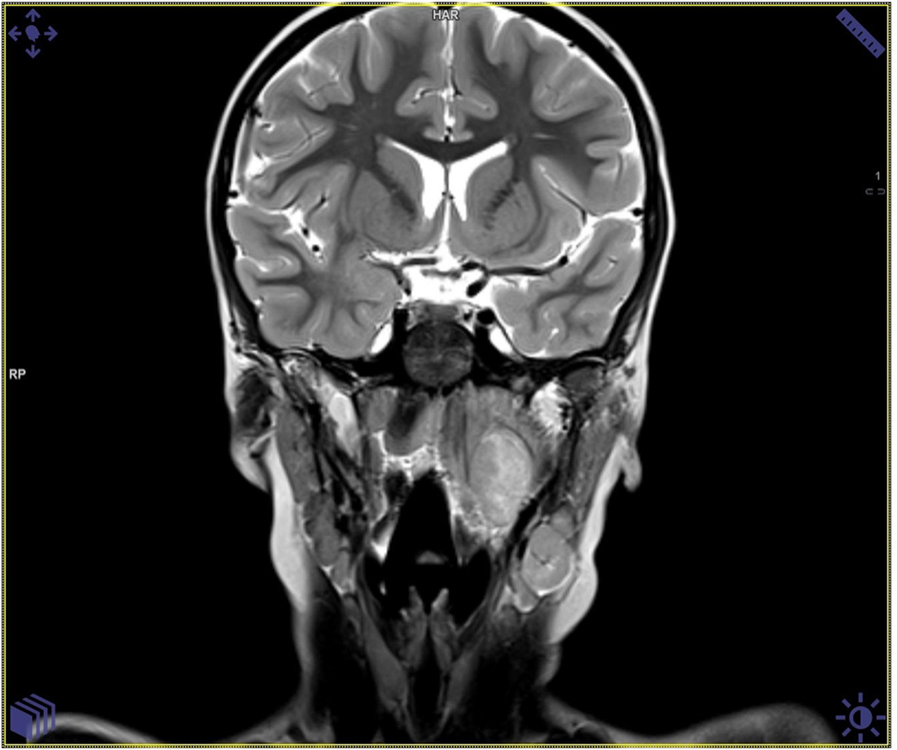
MRI scan of the head and neck of 5-years old child presented the solid mass in the peripharyngeal area.  Sized 20 × 14 × 30 mm lesion modulatesd the left neck vessels and left tonsil
